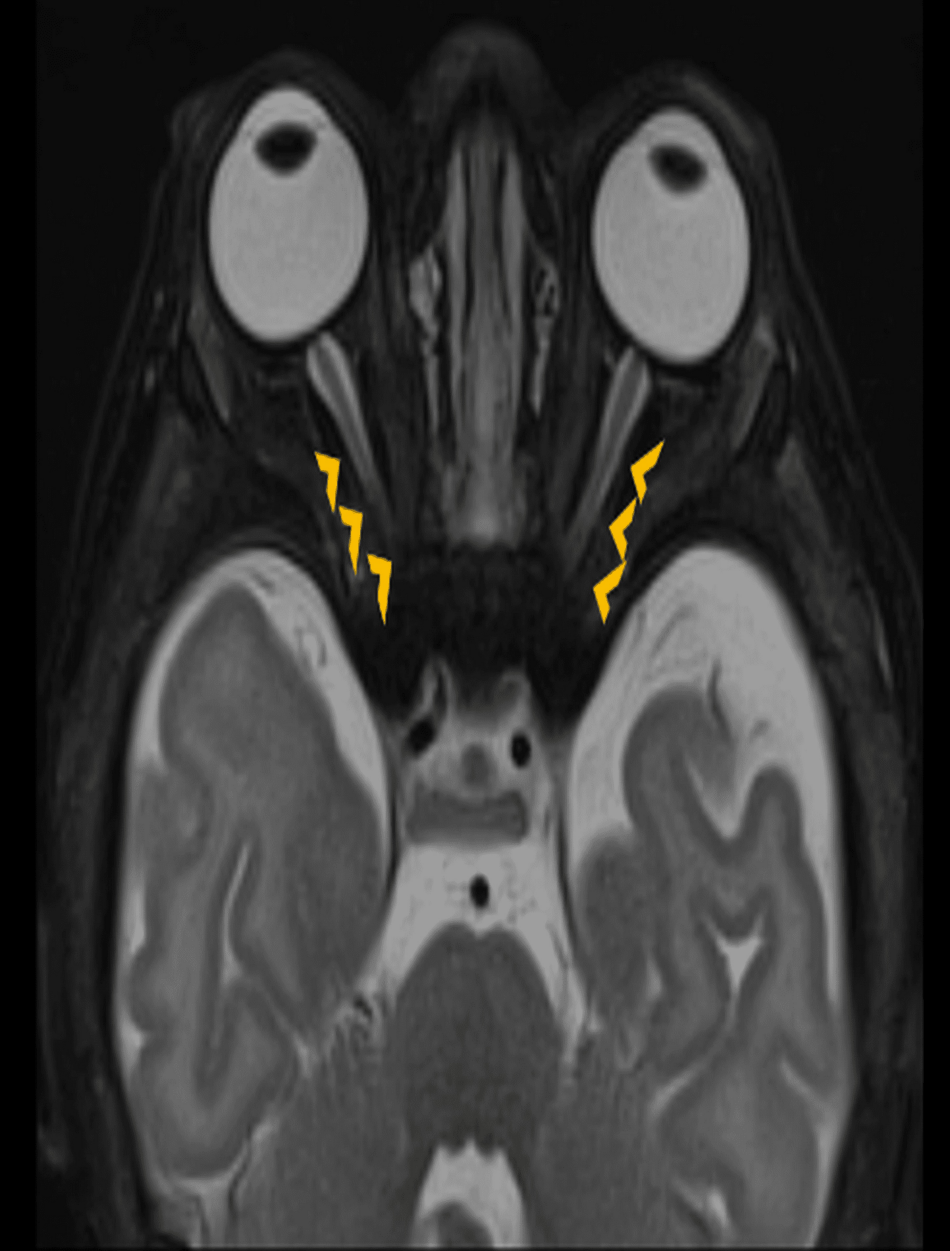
MRI T2 fat saturated sequence in axial section shows thinning of bilateral optic nerves (arrow heads), right more than left
